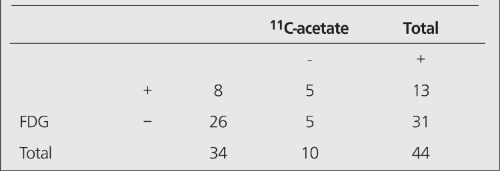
Matrix analysis showing number of positive PET examinationsusing FDG and ^11^C-acetate in 44 patients with hepatocellularcarcinoma (HCC). For statistical analysis (c.f. Results) McNemar’s testwas used
